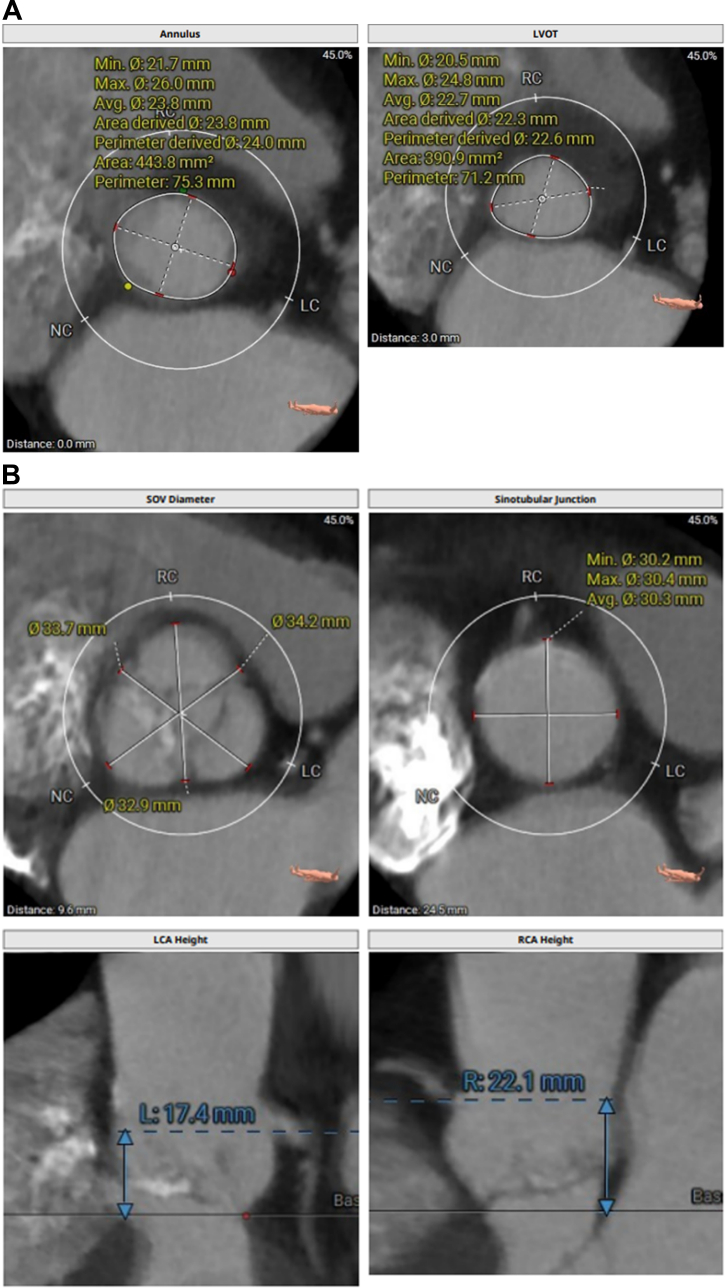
CTA Images (A and B) Index CTA images with annular and LVOT measurements. CTA = computed tomography angiography; LC = left coronary cusp; LVOT = left ventricular outflow tract; NC = non coronary cusp; RC = right coronary cusp.
